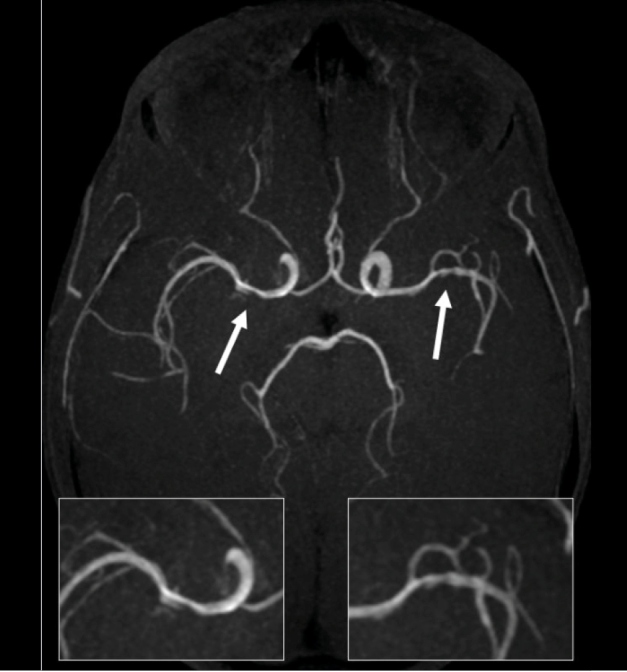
Time-of-flight angio-MRI sequence demonstrating irregular stenosis (white arrows) along medium cerebral arteries.
